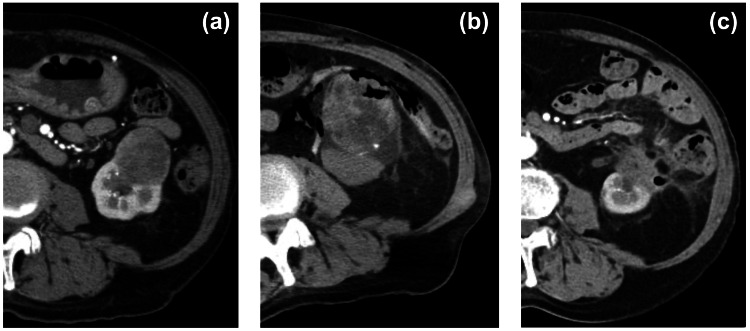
Case 2: Delayed renal abscess associated with bowel injury (a) Contrast-enhanced CT showing a 42 mm papillary renal cell carcinoma in the right kidney. (b) CT obtained four weeks later revealing a renal abscess adjacent to the ablation zone. (c) Follow-up CT obtained three months later showing improvement of the renal abscess.
